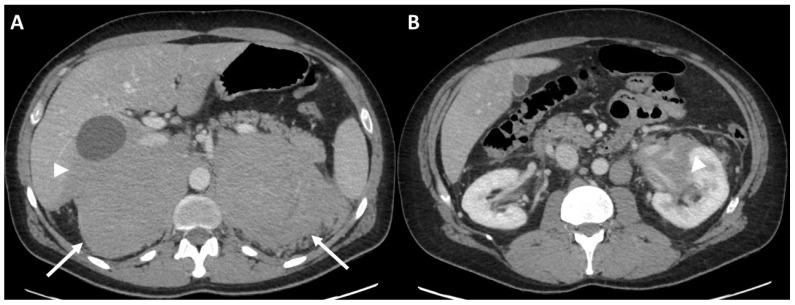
Case #1. Baseline CT images (A = bilateral adrenal lesion; B = left kidney invasion). The arrows showed the adrenal lesions. The arrowhead showed the invasion in the left renal pelvis.
